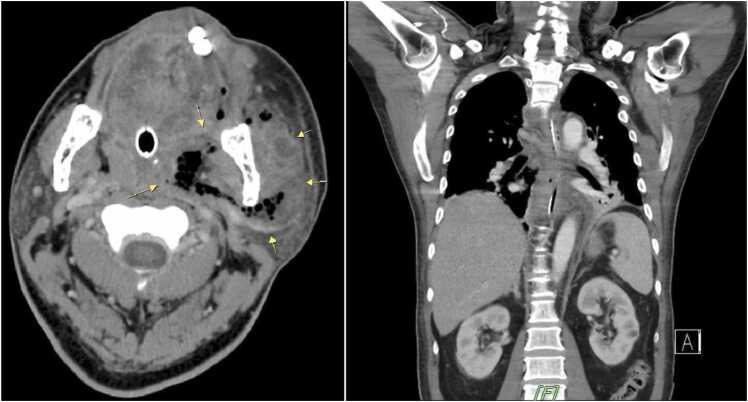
Initial CT scan image revealed abscess formation at left masticator space and palatine tonsil (yellow arrow), air-containing and fat stranding at middle and posterior mediastinum surrounding trachea, high suspected descending necrotizing mediastinitis(B), and bilateral basal lung atelectasis and empyema.
